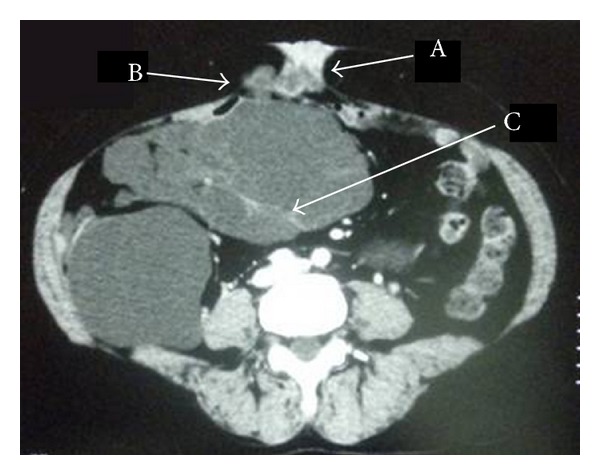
Computed tomography images of the BMPM: the arrows indicate the incisional hernia (A), multicystic mass surrounded to umbilicus (B), and intraabdominal extension (C).
